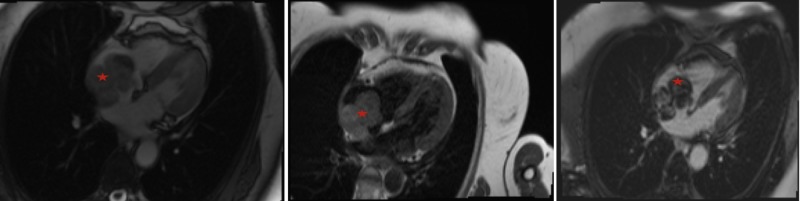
Cardiac MRI imaging of the right atrial mass. Left, SSFP; middle, T1-weighted; right, delayed enhancement images. A bilobed mass (asterisk) was attached to the right atrial wall. The mural component measured 2.3 cm x 4.3 cm x 3.7 cm (TR by AP by cc) and was homogeneously hyperintense to myocardium on T1- and T2-weighted imaging. The mobile, blood pool component of the mass measured 3.1 cm x 4.3 cm x 3.9 cm and was more heterogeneous on T1 than the mural component. The mass demonstrated heterogeneous enhancement on delayed enhancement images. During atrial contraction, the mass appeared to deform the tricuspid valve towards the ventricular cavity.
